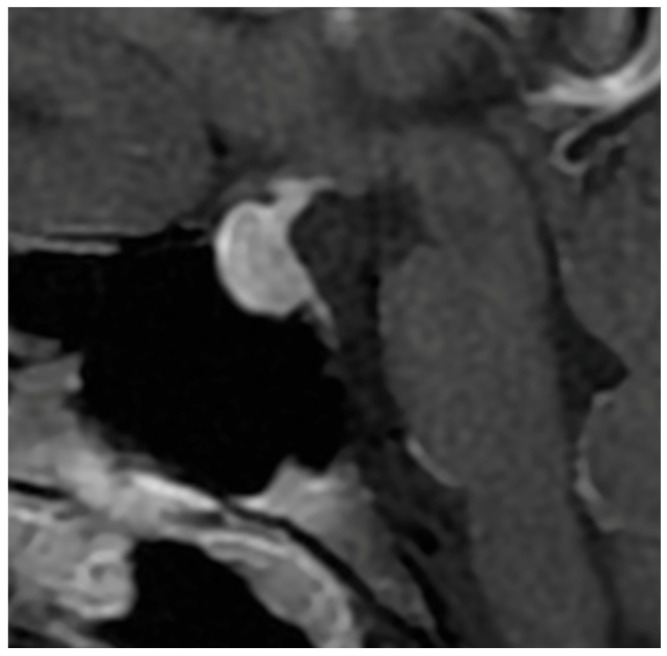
Sagittal T1-weighted image after Gadolinium injection showing a homogeneous enhancement of the pituitary mass in the case of hypophysitis.
